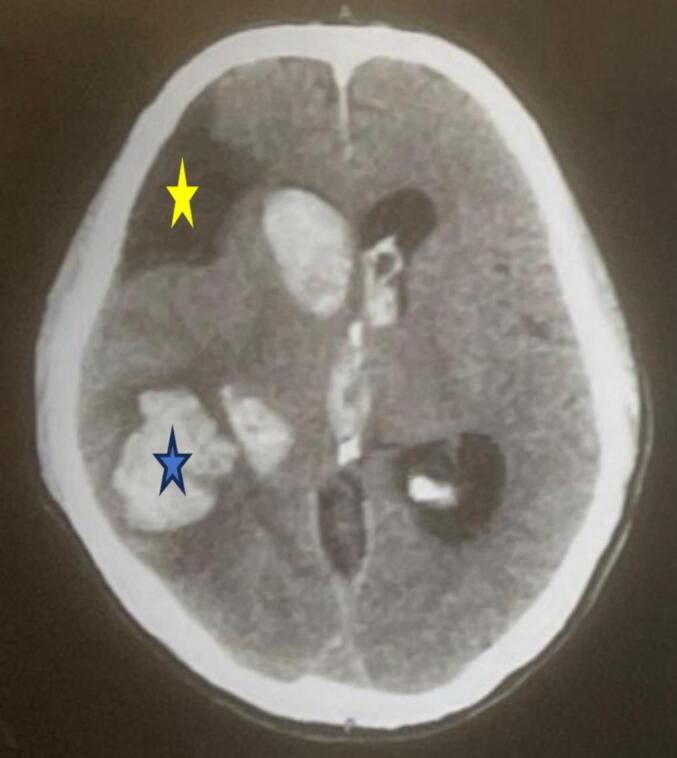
A non-contrast head CT scan shows right parietal intracerebral hematoma with intraventricular extension (blue star) with old infarct over right frontal region (yellow star).
